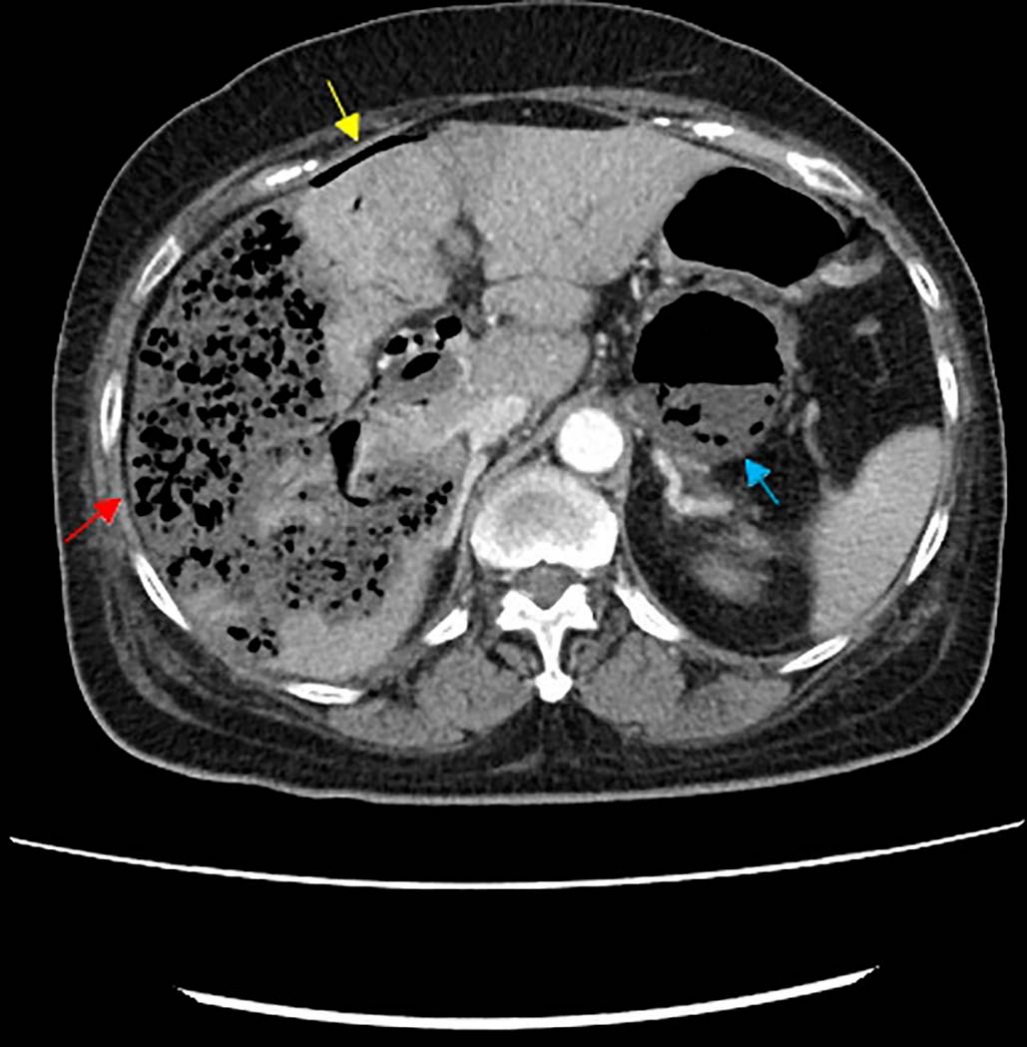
Abdominal computed tomography demonstrated multifoci of liver necrosis with gas-forming abscesses (red arrow), pneumoperitoneum (yellow arrow), and retroperitoneal abscesses (blue arrow).
